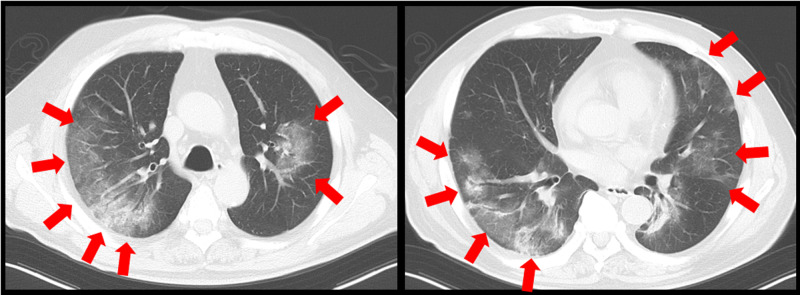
Chest computed tomography scans taken at admission
